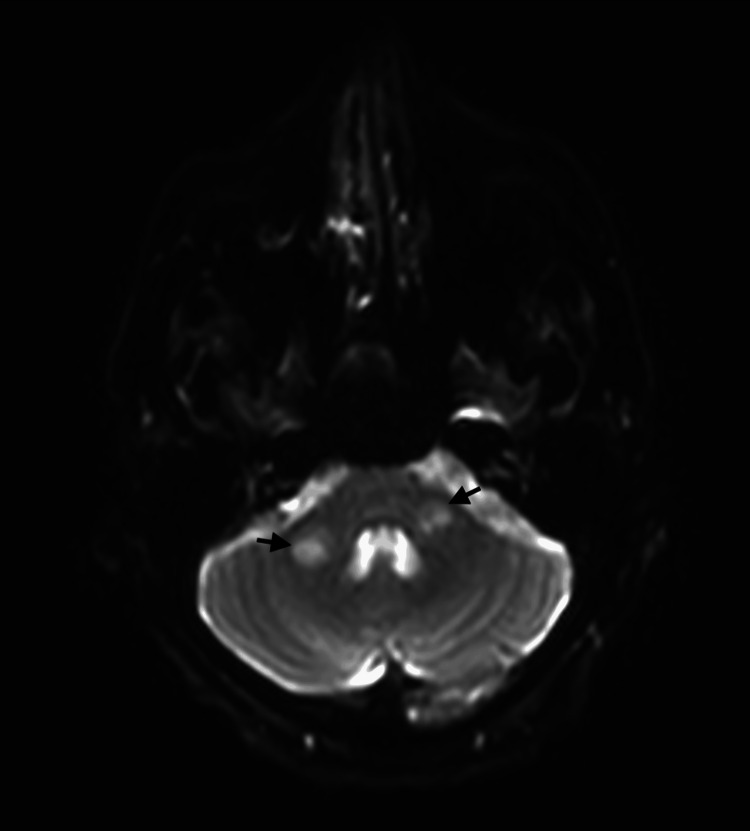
MRI showing subacute brainstem and middle cerebellar peduncle infarcts (arrows).
